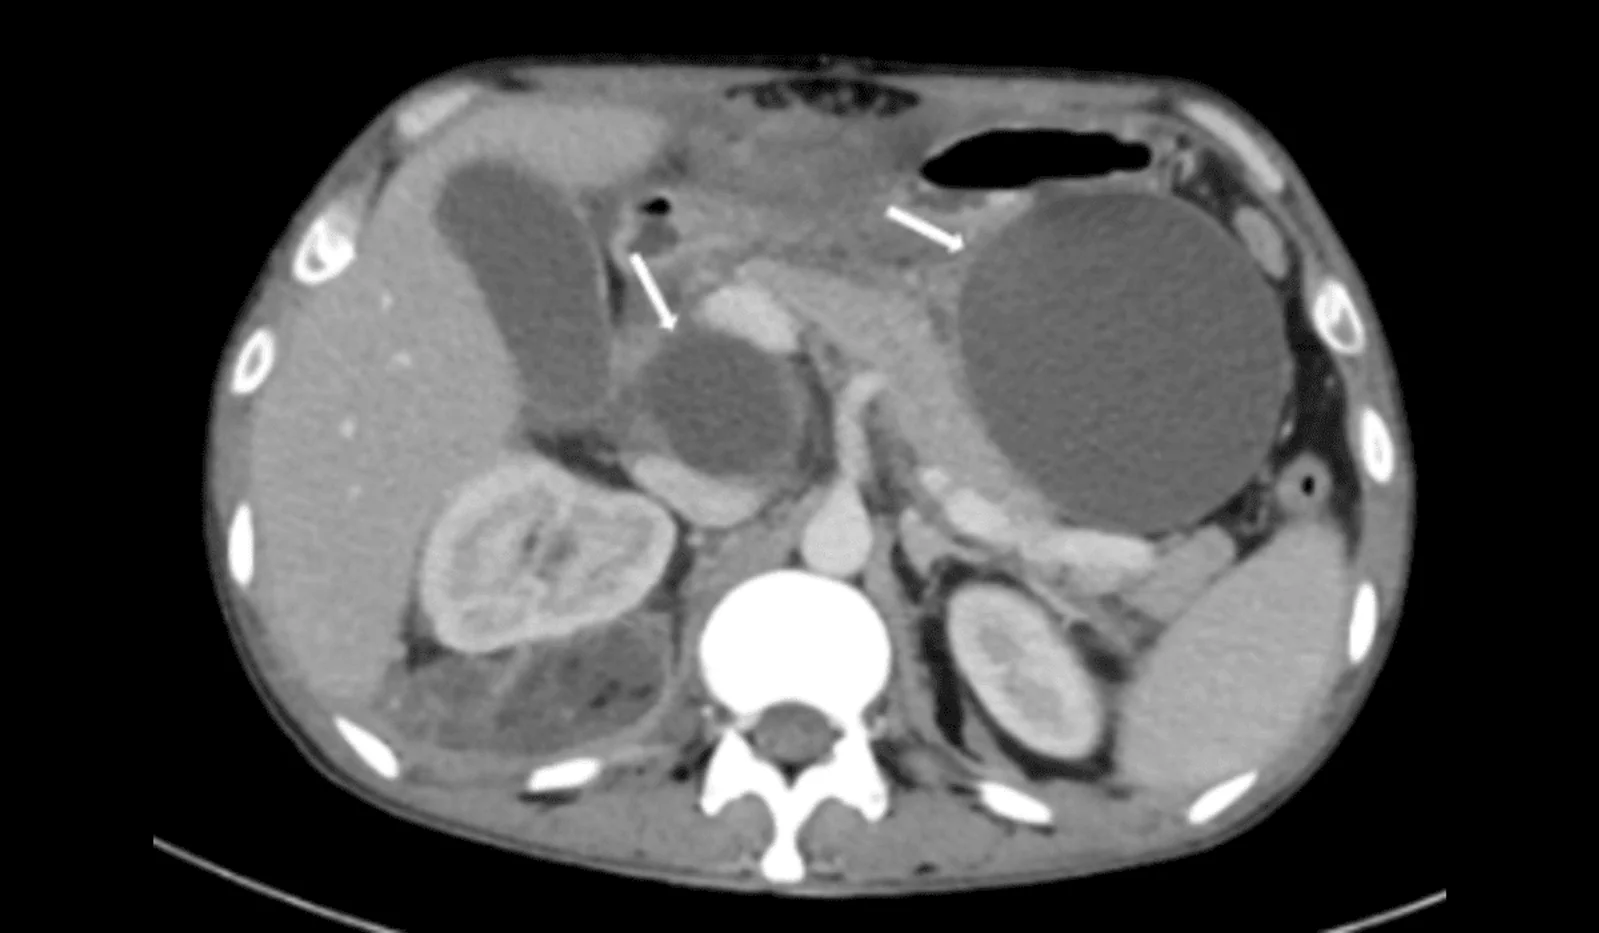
Axial section of contrast-enhanced CT of the abdomen shows a few, well-defined, peripherally enhancing collections adjacent to the pancreatic head and tail, suggestive of pancreatic pseudocysts (white arrows)
